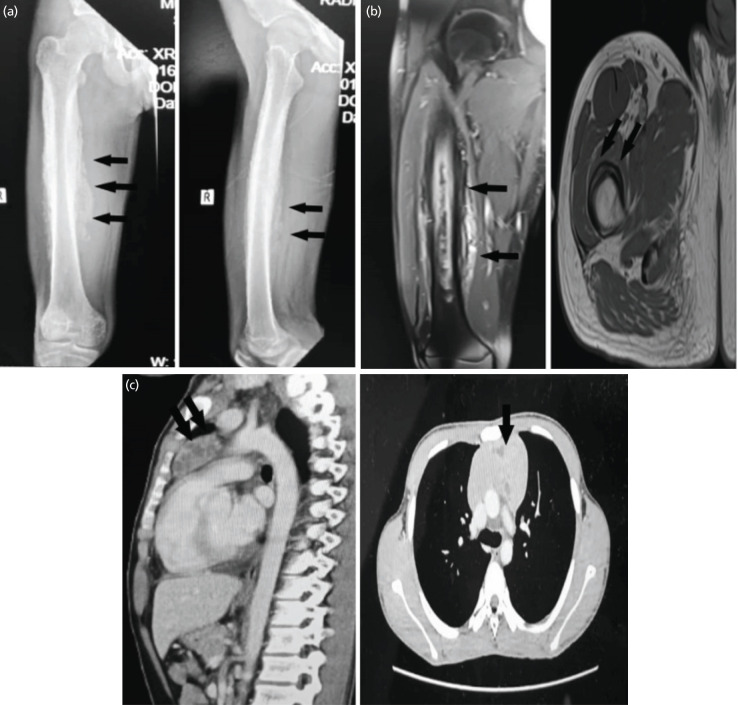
(a) Radiograph showing lamellated periosteal reaction along the diaphysis of the femur (arrow). (b) MRI showing features of the malignant bone tumour (arrow). (c) CT Thorax showing anterior mediastinal soft tissue mass (arrow).
